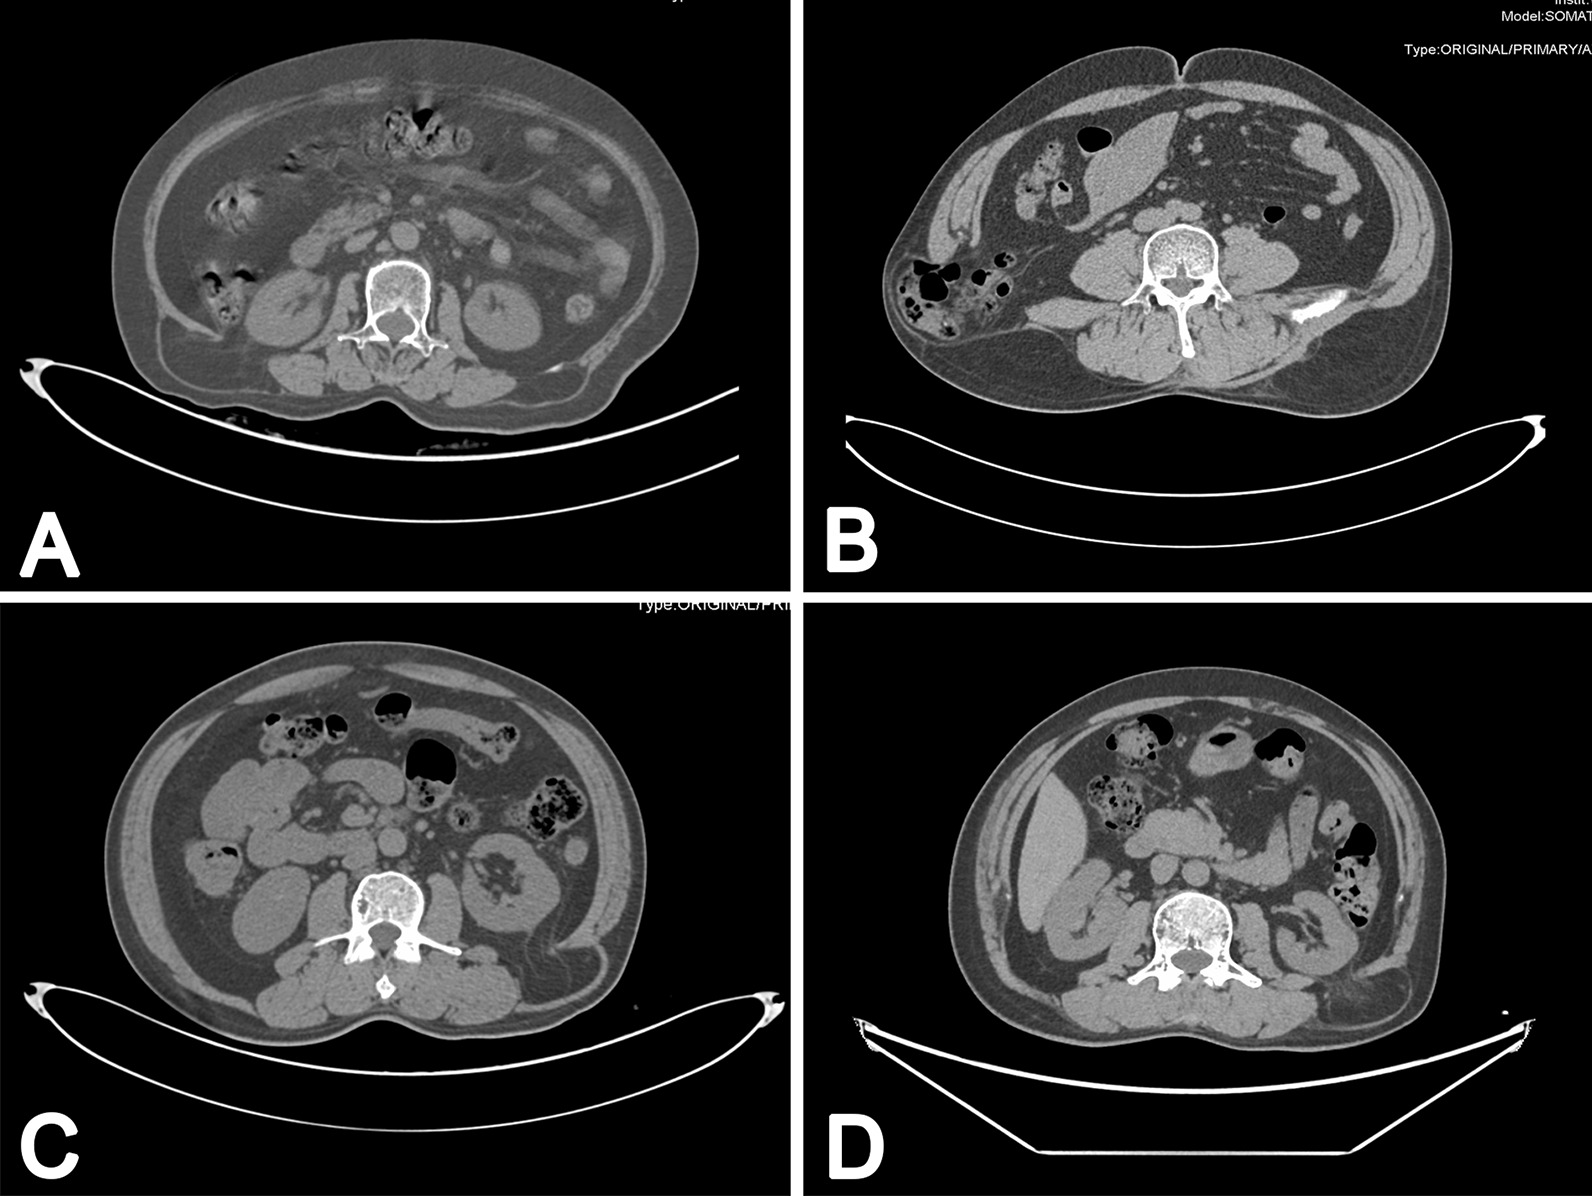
Abdominal CT showing the hernia content. A the bilateral lumbar hernia was showed; B CT demonstrating herniation of part of ascending colon bowel through a right abdominal wall defect; C and D CT showing local abdominal fat herniation into subcutaneous fat layer in the left lumbar back
